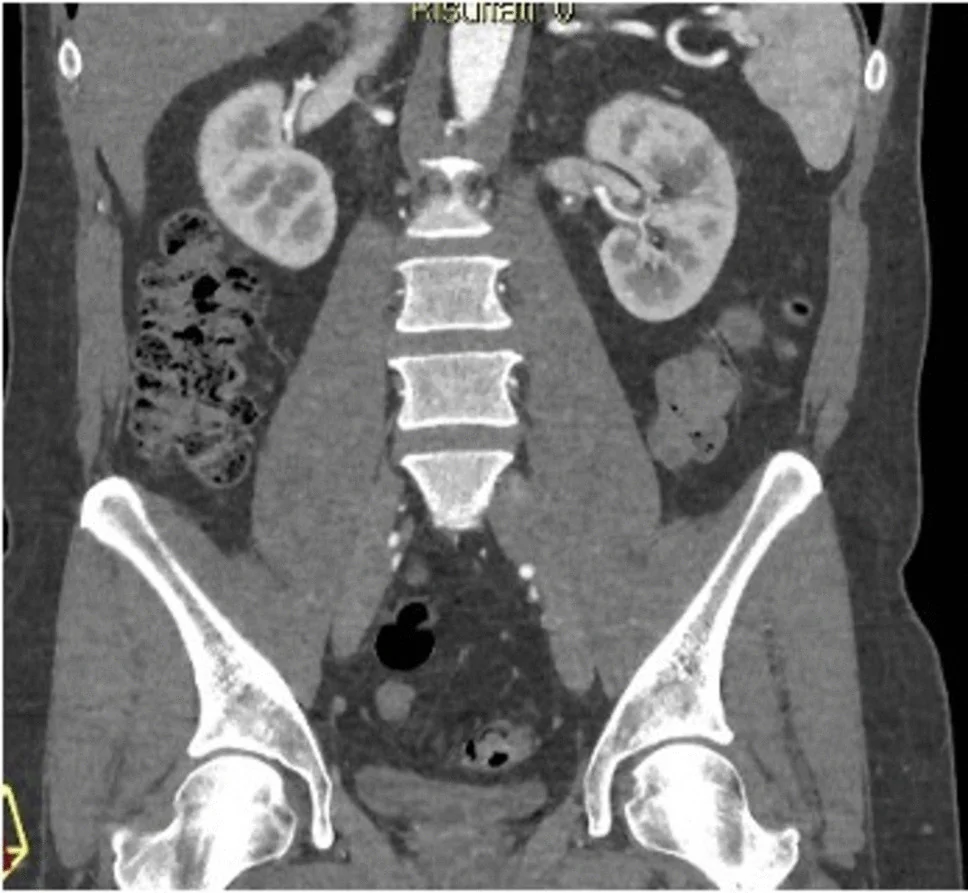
One-month CT scan: no longer detectable hypodense thickening of left renal artery
